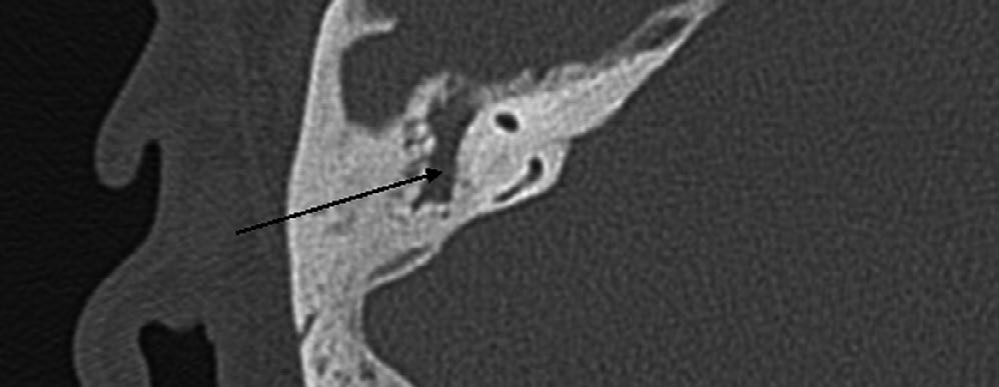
Axial temporal bone CT views of opacification extending into mastoid cavity (see line arrow)
